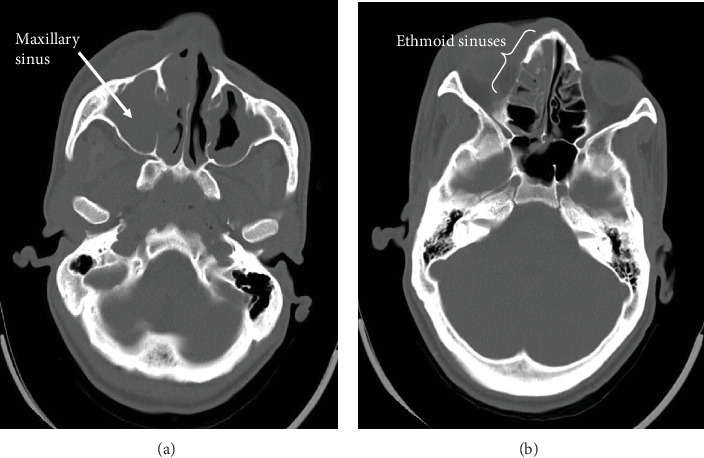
Axial slices of postcontrast CT of the brain and sinuses viewed on bony window: complete opacification of the right (a) maxillary sinus, with associated opacification of the anterior and middle (b) ethmoid sinuses. No bony erosion or sclerotic changes were observed.
